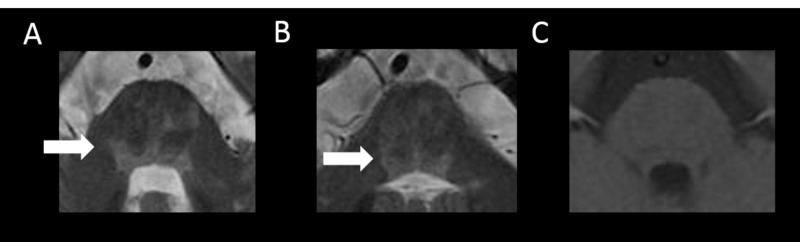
Brain MRI findings - lesions in the brainstem A, B: brain T2-weighted imaging shows multiple high-intensity lesions in the brainstem, including the intramedullary running path of the trigeminal nerve (white arrows); C: the lesions exhibit no gadolinium enhancement MRI: magnetic resonance imaging
